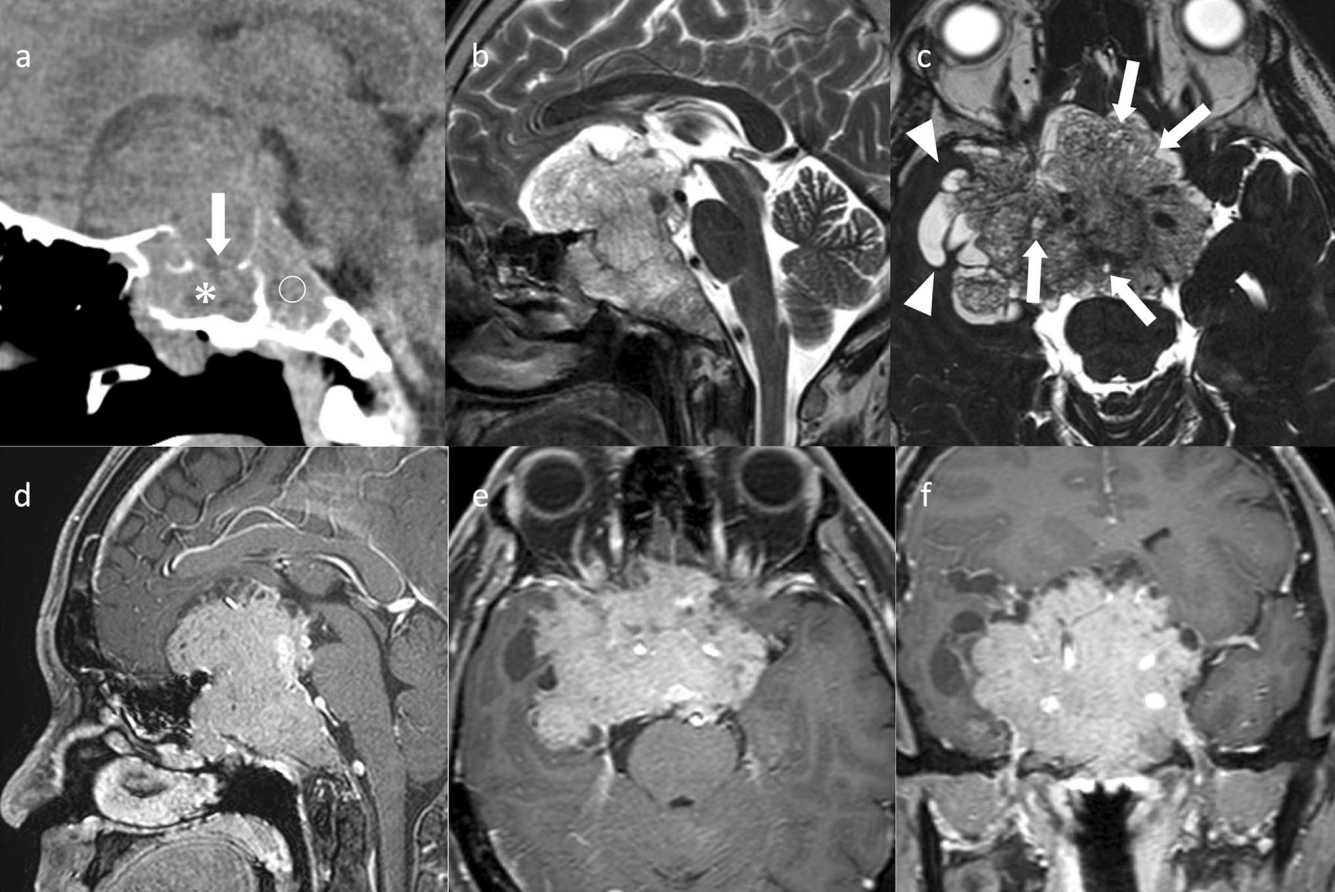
Silent corticotroph PitNET/pituitary adenoma. A male patient in his 40 s, with diplopia, disorientation, and cortisol levels within normal range. a Noncontrast CT shows destruction of the sella turcica (arrow) and invasion of the sphenoid sinus (asterisk) and the clivus (open circle). b Sagittal T2WI shows a mass with hyperintensity and iso-intensity areas. c Axial heavy T2WI shows small multiple cysts within the mass. Cysts around the mass likely represent trapped encysted pools of cerebrospinal fluid. d–f Contrast-enhanced T1WI shows a large mass centered in the sella turcica and invading the surrounding areas (the bilateral cavernous sinuses, and inferiorly, the sphenoid sinus and the clivus)
